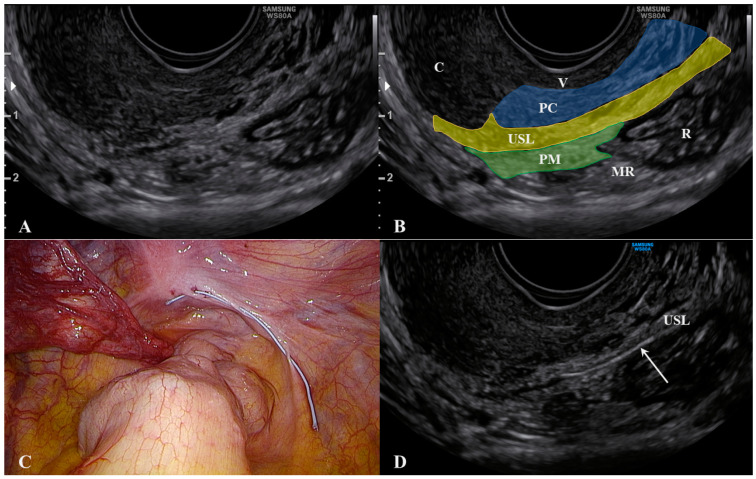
Ultrasound visualization of the uterosacral ligament, paracervix, and parametrium. Figure (A) shows the normal appearance and explanations are reported in figure (B). A polyester suture was placed on a physiological USL during laparoscopy (figure (C) and identified via ultrasound (arrow) in figure (D). Abbreviations: C, cervix; MR, mesorectum; PC, paracervix; PM, parametrium; R, rectum; USL, uterosacral ligament; V, vagina wall.
